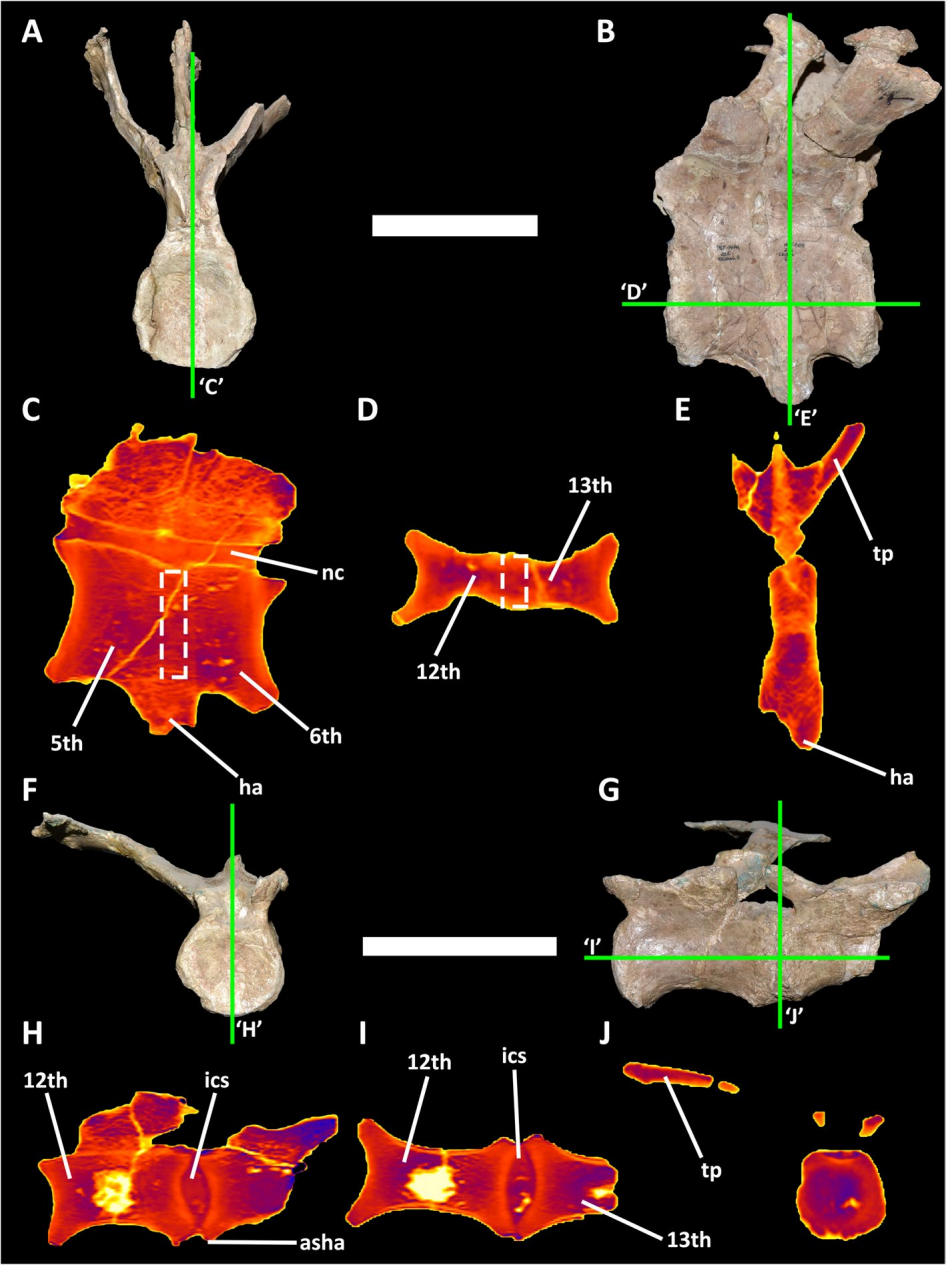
CT-scans of pathologic and normal caudal vertebrae of *Aucasaurus garridoi* MCF-PVPH-236. A, anterior; and B, lateral views of the 5th and 6th caudal vertebrae, and 5th haemal arch. Tomographic images in C, parasagittal section; D, coronal section; and E, transverse section. F, anterior; and G, lateral views of the 12th and 13th caudal vertebrae. Tomographic images in H, parasagittal section; I, coronal section; and J, transverse section. The dashed-line boxes indicate the zone among fused vertebrae without an intercentrum space. The green lines indicate the planes of the slices. Abbreviations: 5th, fifth caudal vertebra; 6th, sixth caudal vertebra; 12th, twelfth caudal vertebra; 13, thirteenth, caudal vertebra; asha, articulation surface for the haemal arch; ha, haemal arch; ics, intercentrum space; nc, neural canal; tp, transverse process. Scale bars 10 cm
